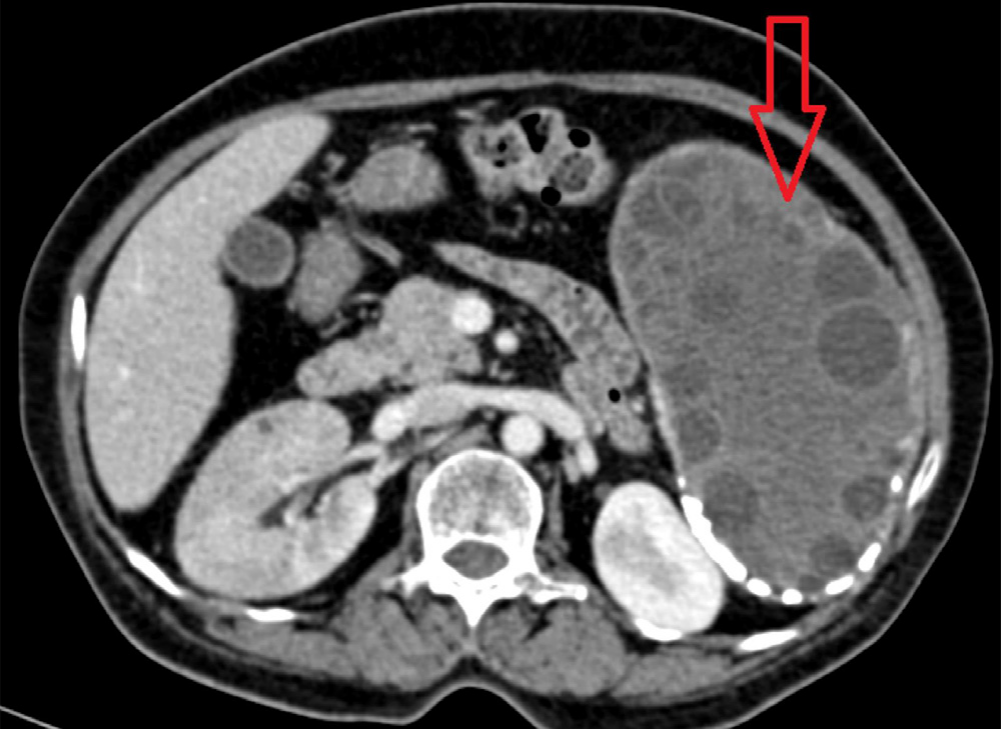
CT scan axial window showing multiloculated hypodense cyst multiple peripherally located daughter cysts. Wall of the lesion was partially calcified. The lesion measured 14 × 7.7 × 13.2cm.
